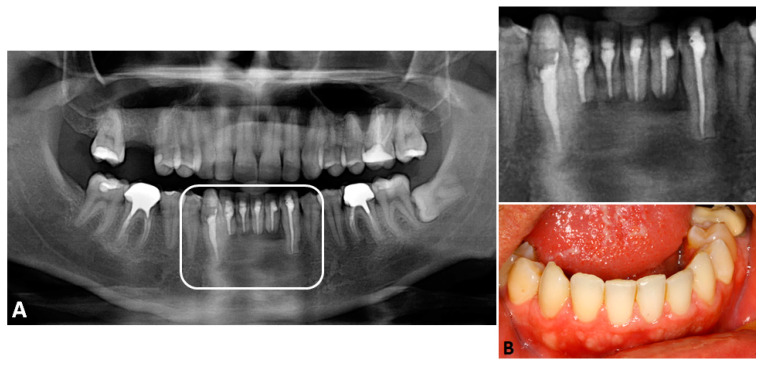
Follow-up of fifteen months after the second procedure. (A)—The panoramic radiograph showed bone neoformation within the entire surgical site, with no signs of recurrence. (B)—Clinically, the mucosa was found to be healthy, and the involved teeth were in function, without functional or aesthetic repercussions.
